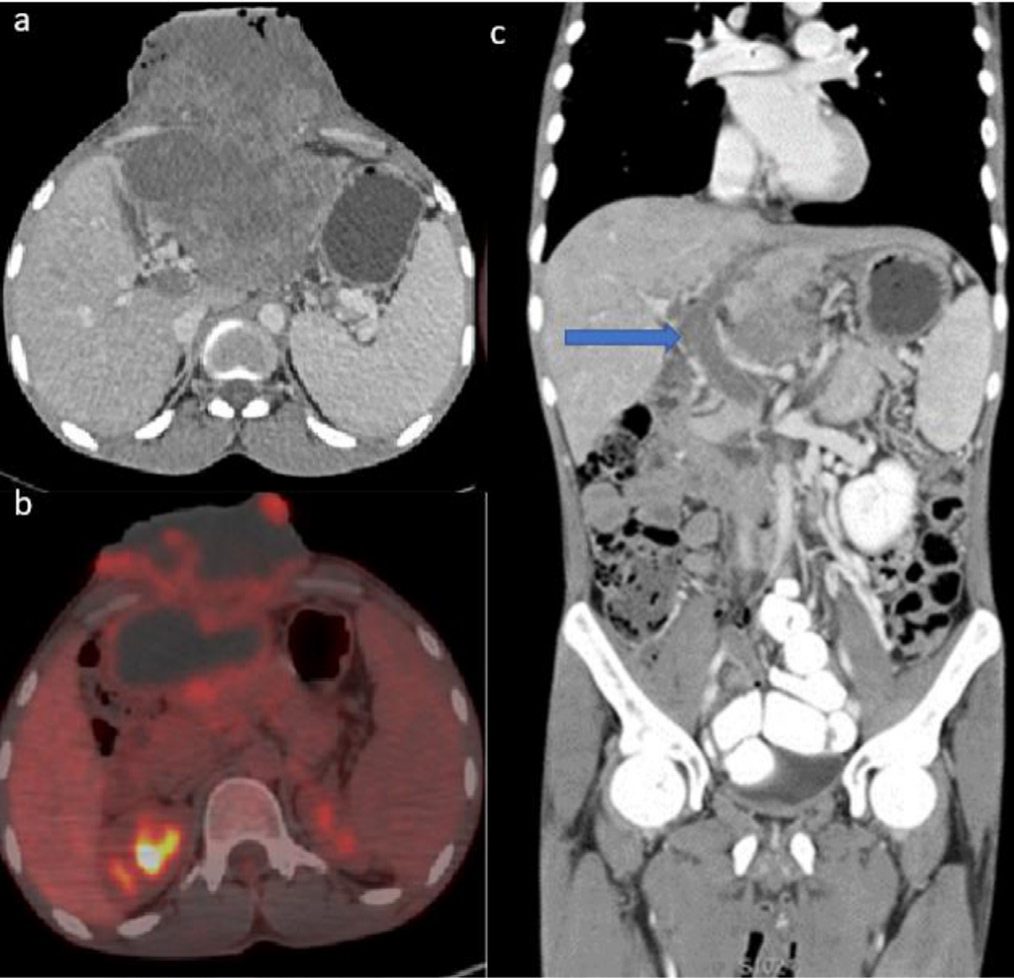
Axial porto-venous CT (a) and axial-fused FDG PET CT (b) images showing a large heterogenous left lobe of the liver mass invading the anterior abdominal wall. Coronal portovenous phase CT image (c) showing a thrombus in the main portal vein (arrow) and left portal vein.
